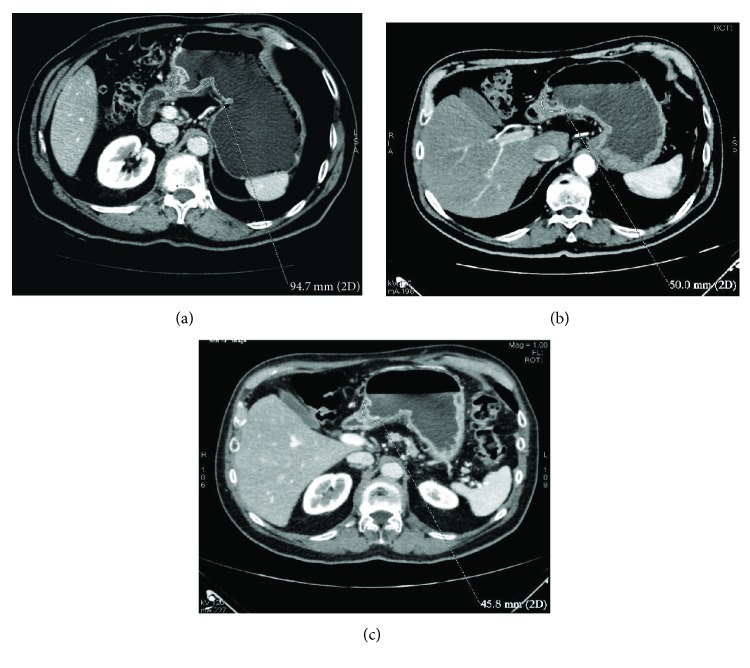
(a–c) Comparison between staging CT (a), intermediate (b) and presurgical (c) CTs in a 71-year-old man with GC (intestinal histotype) located in the antrum and body, along the lesser curvature. It was clear how D-max reduction rate was greater after 2 cycles of chemotherapy ((a) versus (b), 47.2%) than in the remainder of the treatment ((b) versus (c), 10%).
